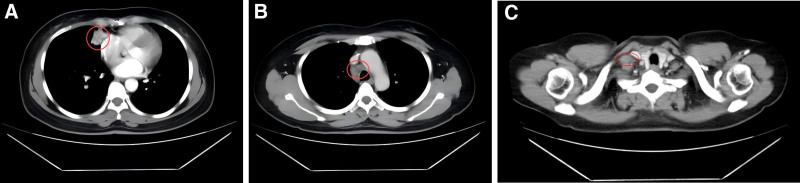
Chest CT follow-up on March 17, 2020. (A) Significant reduction in the right lung lesion; (B) reduction in the size of the enlarged mediastinal lymph nodes; (C) reduction in the size of the metastatic lymph node in the right supraclavicular region. CT = computed tomography.
